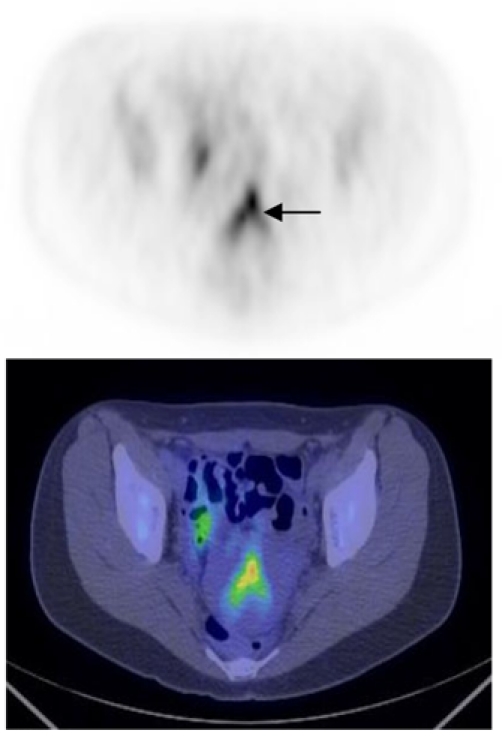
FDG PET CT scan (axial view) of the pelvis. Activity is seen in the endometrial cavity in this patient who was menstruating (arrow). On the fused image, the uterus is seen to be retroverted [Image courtesy of Centre for Molecular Imaging, Peter MacCallum Cancer Centre, East Melbourne, Victoria, Australia].
